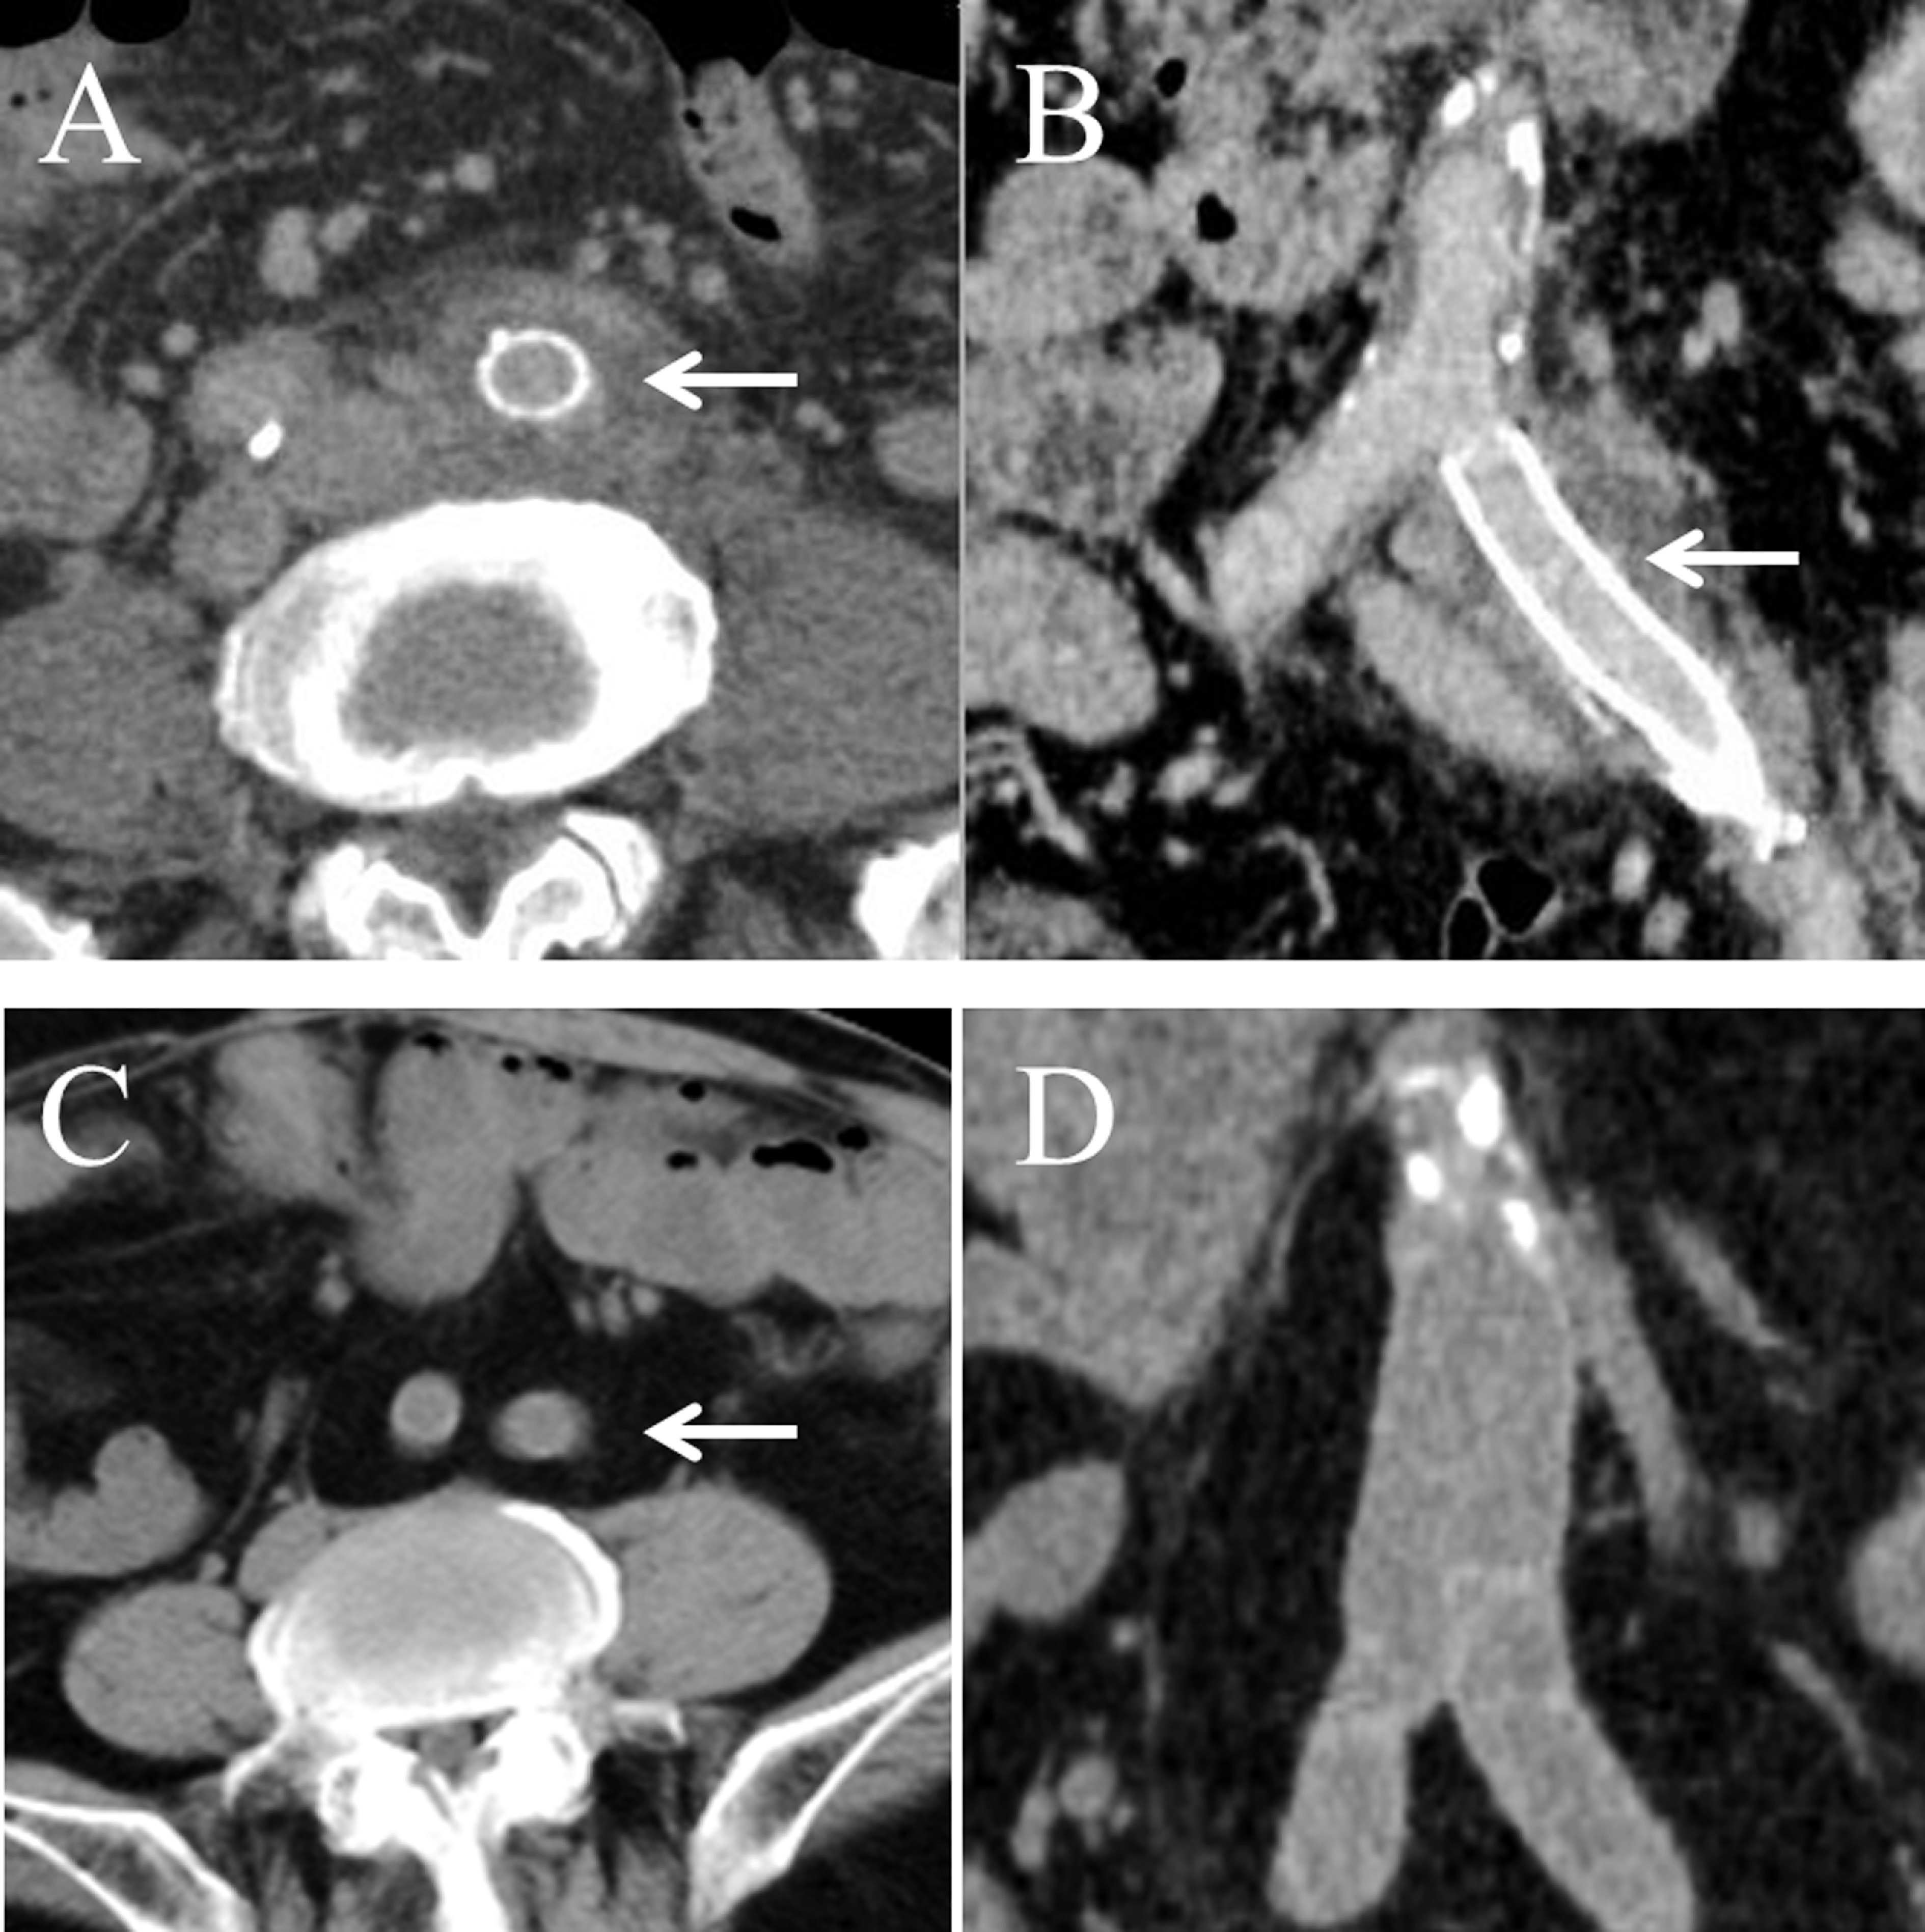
Fig. 1 (A)(B) Preoperative computed tomography (CT) images showing the bare iliac stent (arrows) and infected aneurysm. (C)(D) Postoperative CT images at the 2-year follow-up showing the prosthesis graft with no reinfection. The graft was wrapped by the omental flap (arrow), which is seen as a low density around the graft.
